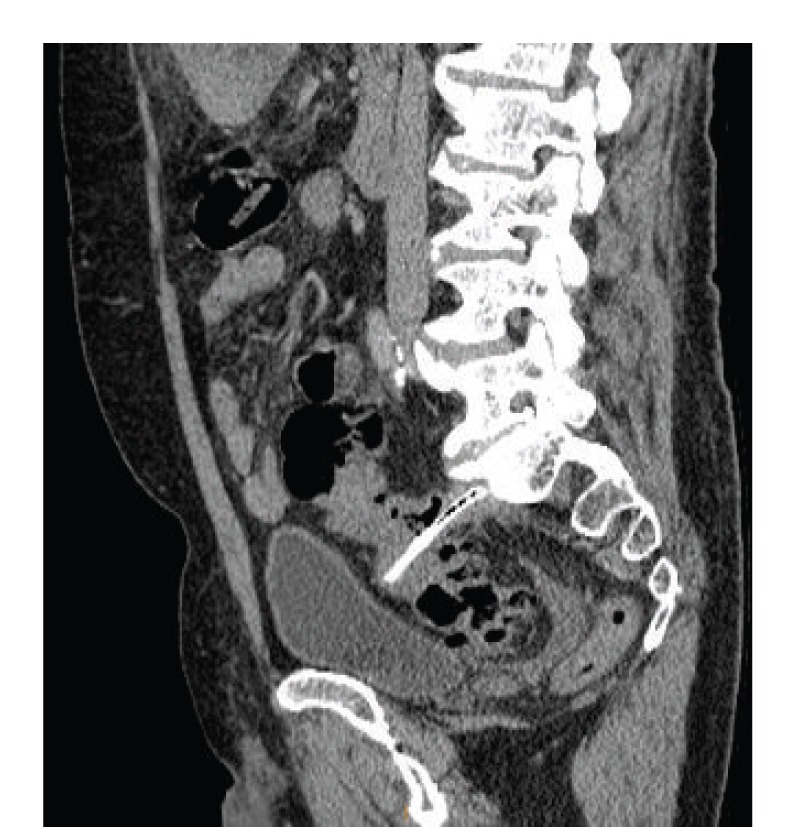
Sigmoid foreign body identified on CT.
